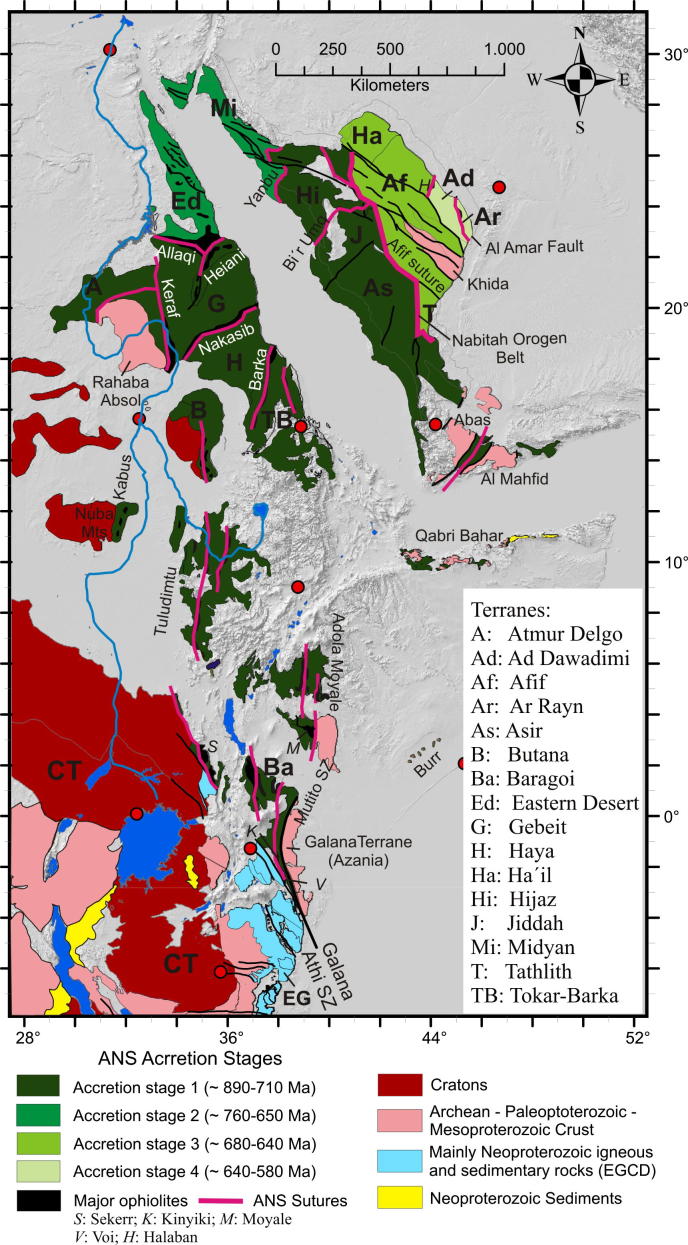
Crustal age domains in the northern East African Orogen and crustal growth phases in the Arabian–Nubian Shield. CT, Congo–Tanzania Craton; EG, Eastern Granulites.
